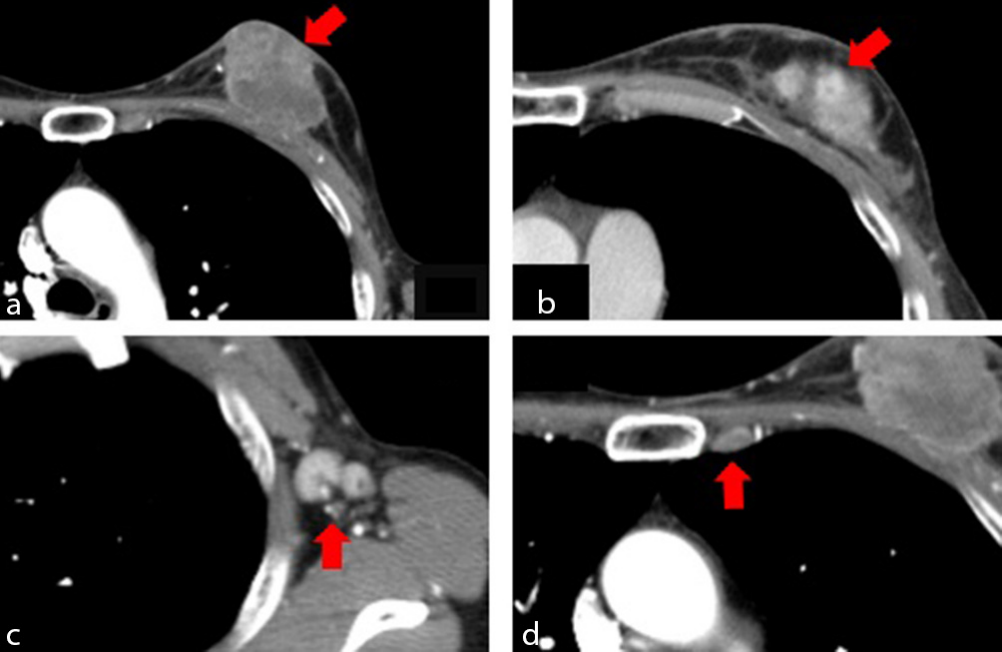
Contrast-enhanced CT images of the patient’s tumour. A 54 mm primary lesion (arrow) is visible on the internal upper area of the left breast, which had invaded the skin (a). A daughter nodule (arrow) visible on the external upper area (b). Enlarged axillary and parasternal lymph nodes (arrows) visible on (c) and (d), respectively.
